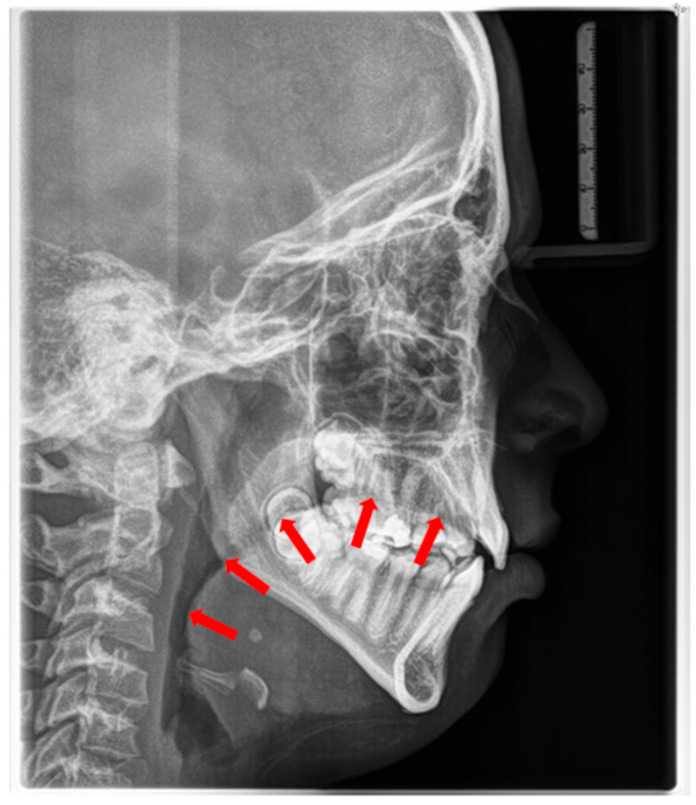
Radiographic cephalogram: Low tongue posture, the tongue protrudes into the oropharynx (red arrows indicate tongue shadow).
